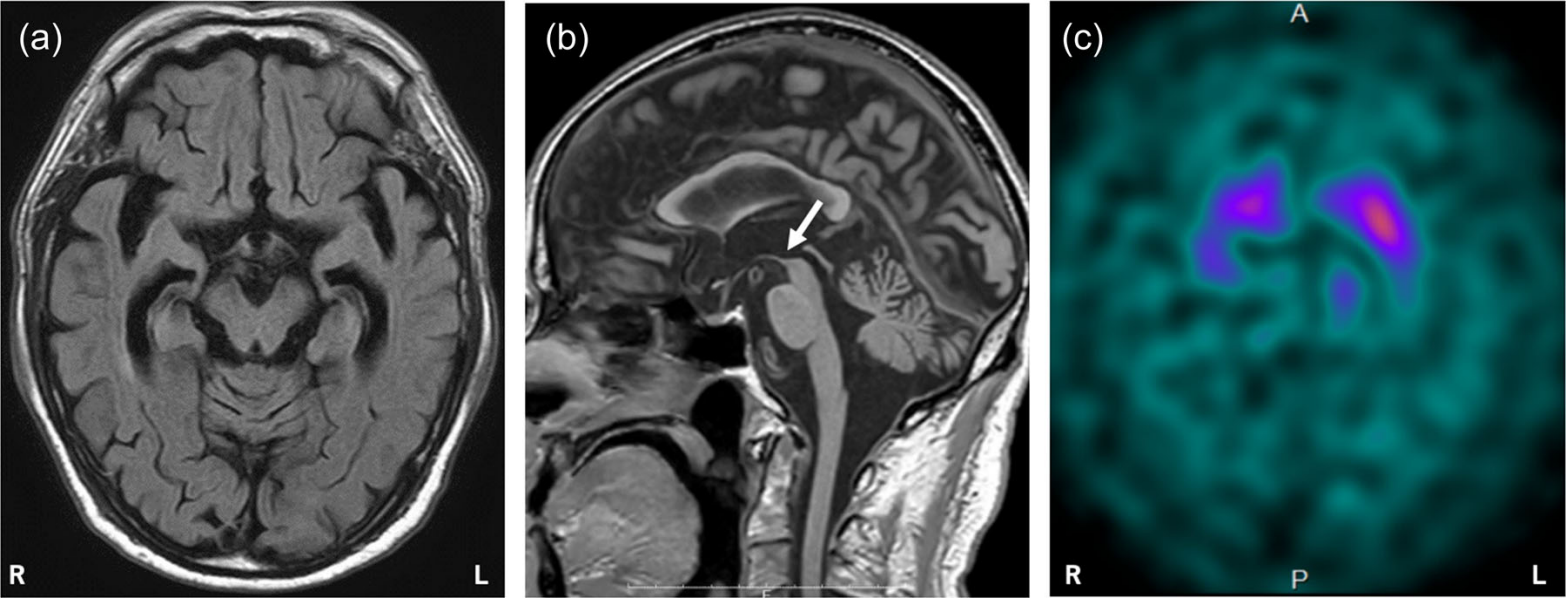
Neuroimaging findings of the patient at the second hospital admission. (a) Axial fluid‐attenuated inversion recovery sequence brain magnetic resonance imaging (MRI) showed mild atrophy of the midbrain and mid‐temporal lobe, including the hippocampus. (b) Sagittal T1‐weighted brain MRI revealed atrophy of the midbrain capsule, which is referred to as the “hummingbird” sign (indicated by the arrow). (c) ^123^I‐ioflupane single‐photon emission tomography shows decreased accumulation in the bilateral striatum.
